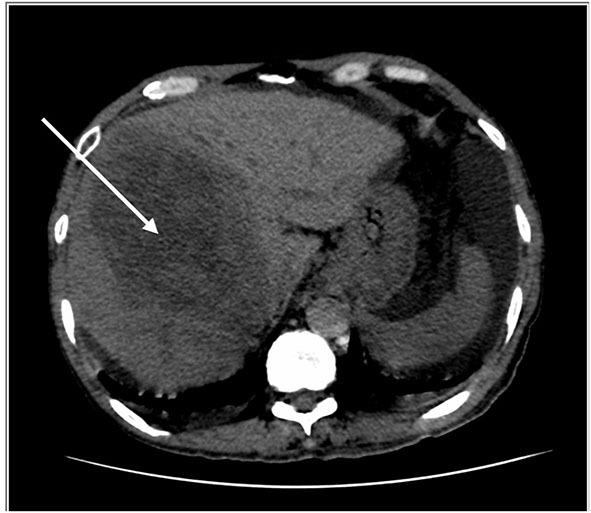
HRCT thorax, including the liver. Key images of two scans of the same patient, at the same slice taken 3 weeks apart. The initial scan shows a large right liver lobe mass (white arrow). Please note that no obvious lesion is seen in the left liver lobe.
